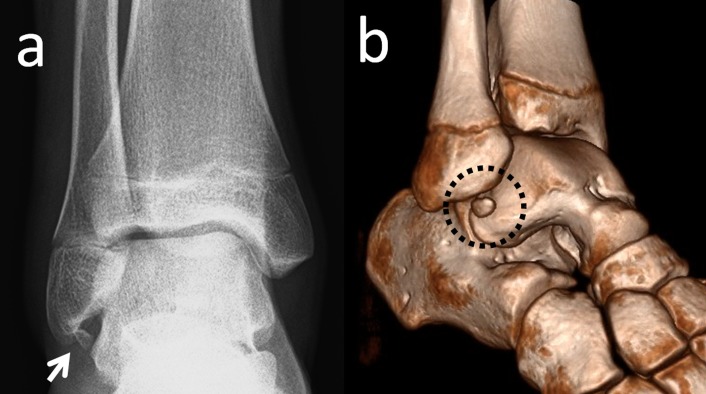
An os subfibulare (a) Anteroposterior foot radiograph, the white arrow is os subfibulare (b) 3D computed tomography (CT) appearance.
